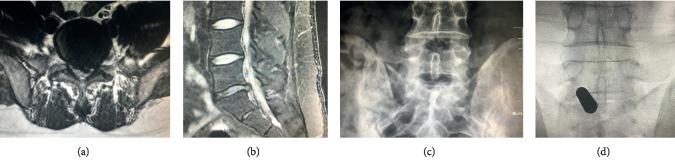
The imaging findings of typical cases of osteotomy in PED. (a) MRI in the coronal plane. (b) MRI in the sagittal plane. (c) Prospective X-ray scan. (d) Intraoperative X-ray scan. The patient in the figure was a 38-year-old man whose height was 180 cm. The herniated nucleus could be identified on the coronal plane of MRI from the medial to the lateral side of the nerve root. Preoperative anterior and posterior X-ray images revealed the width of the interlaminar space, whereas intraoperative X-ray using C-arm fluoroscopy revealed the diameter of the working tube to be considerably larger than that of the interlaminar space.
